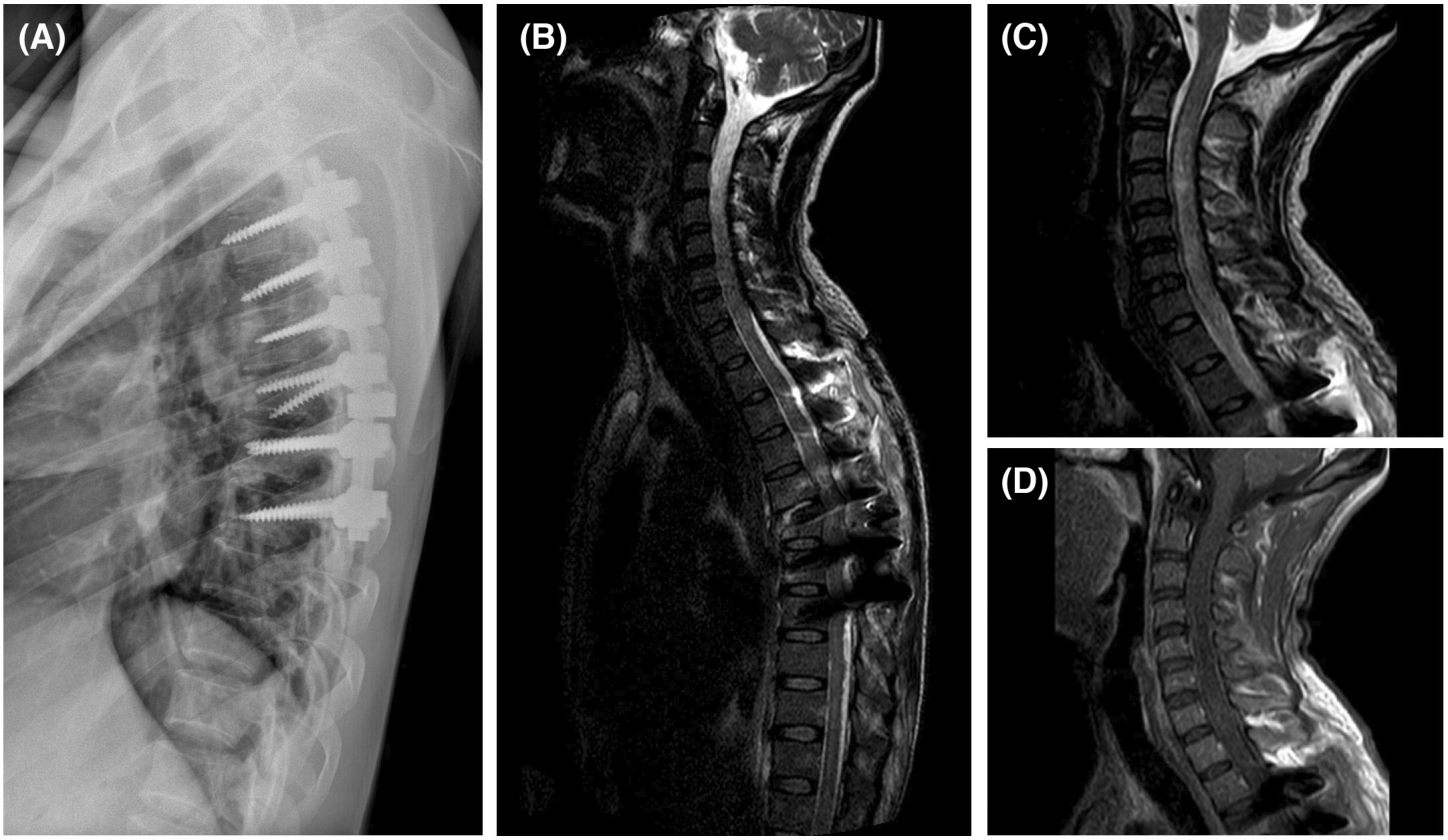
(A) Postoperative plain radiography of the thoracic spine in a lateral view shows Posterior long segment fixation by pedicle screw. (B and C) Sagittal reconstruction T2 weighted images of cervical and thoracic spine MRI on postinjury day 14 show increased signal and swelling between C3 and C7. Artifact from spinal instrumentation obscured visualization of cord segments in midthoracic region (D)
